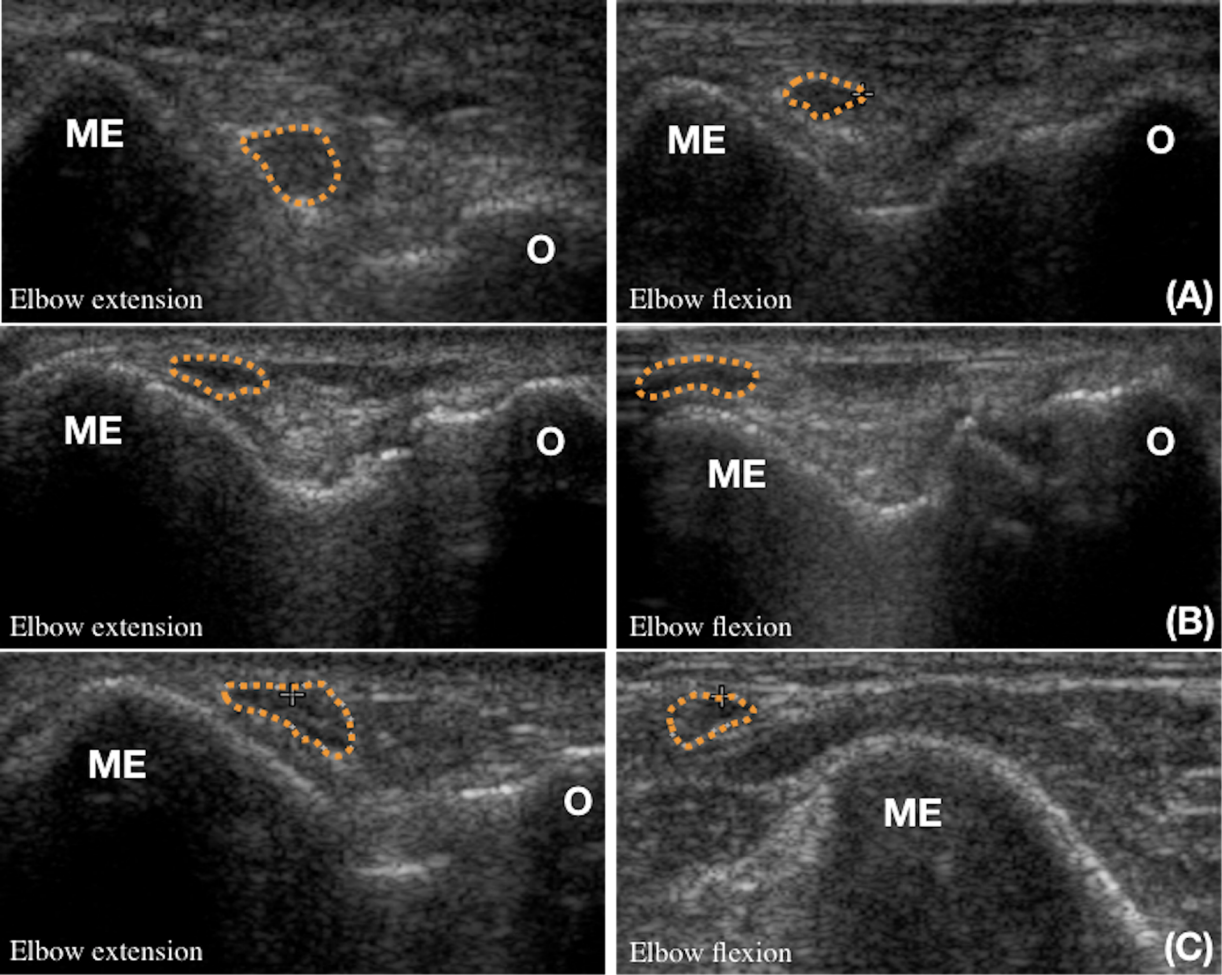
The ultrasonographic classifications of ulnar nerve (dotted line) displacement during elbow flexion. (A) Non-dislocation of ulnar nerve. (B) Partial dislocation of ulnar nerve. (C) Complete dislocation of ulnar nerve. ME, medial epicondyle; O, olecranon.
